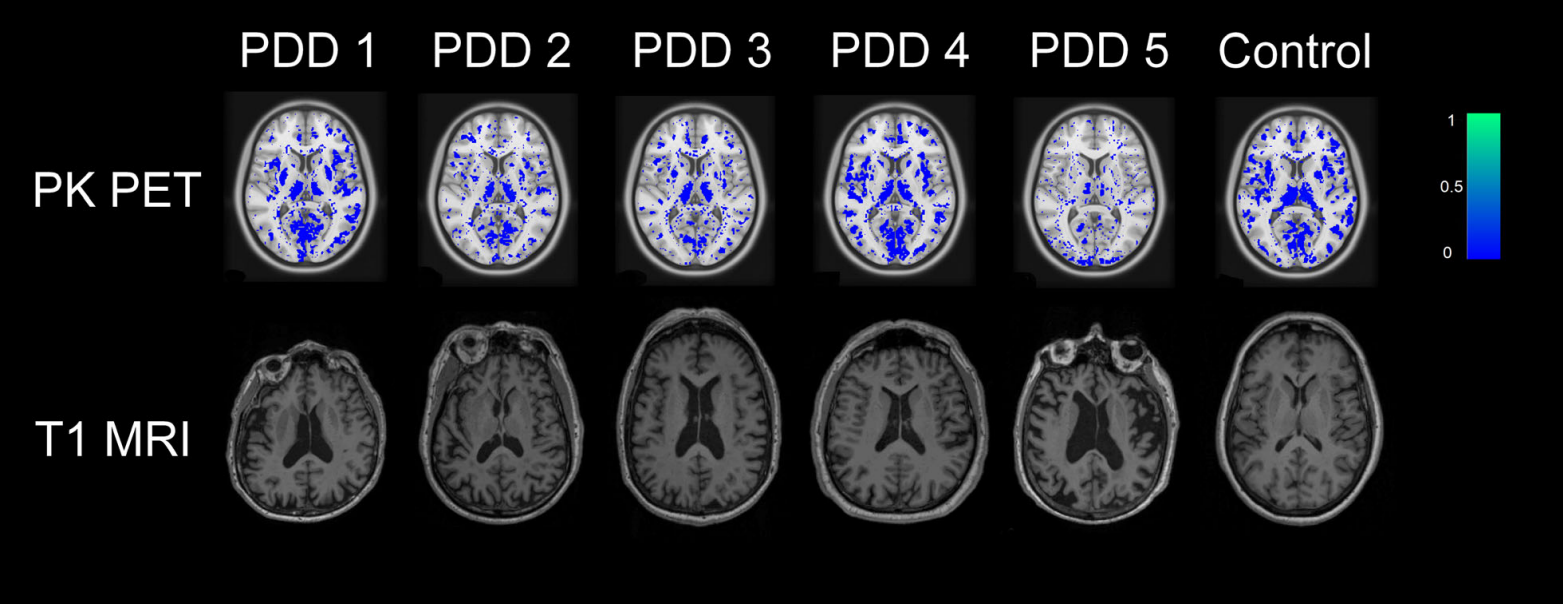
Axial ^11^C‐PK11195 BP_ND_ images and T1‐weighted structural MRI of the five PDD included in the study and one representative control subject (65‐year‐old female participant, ACER 96/100). PDD patients have similar ^11^C‐PK11195 binding than the control participants, except PDD 5 who has lower binding values in several regions (cf. Table 1).
